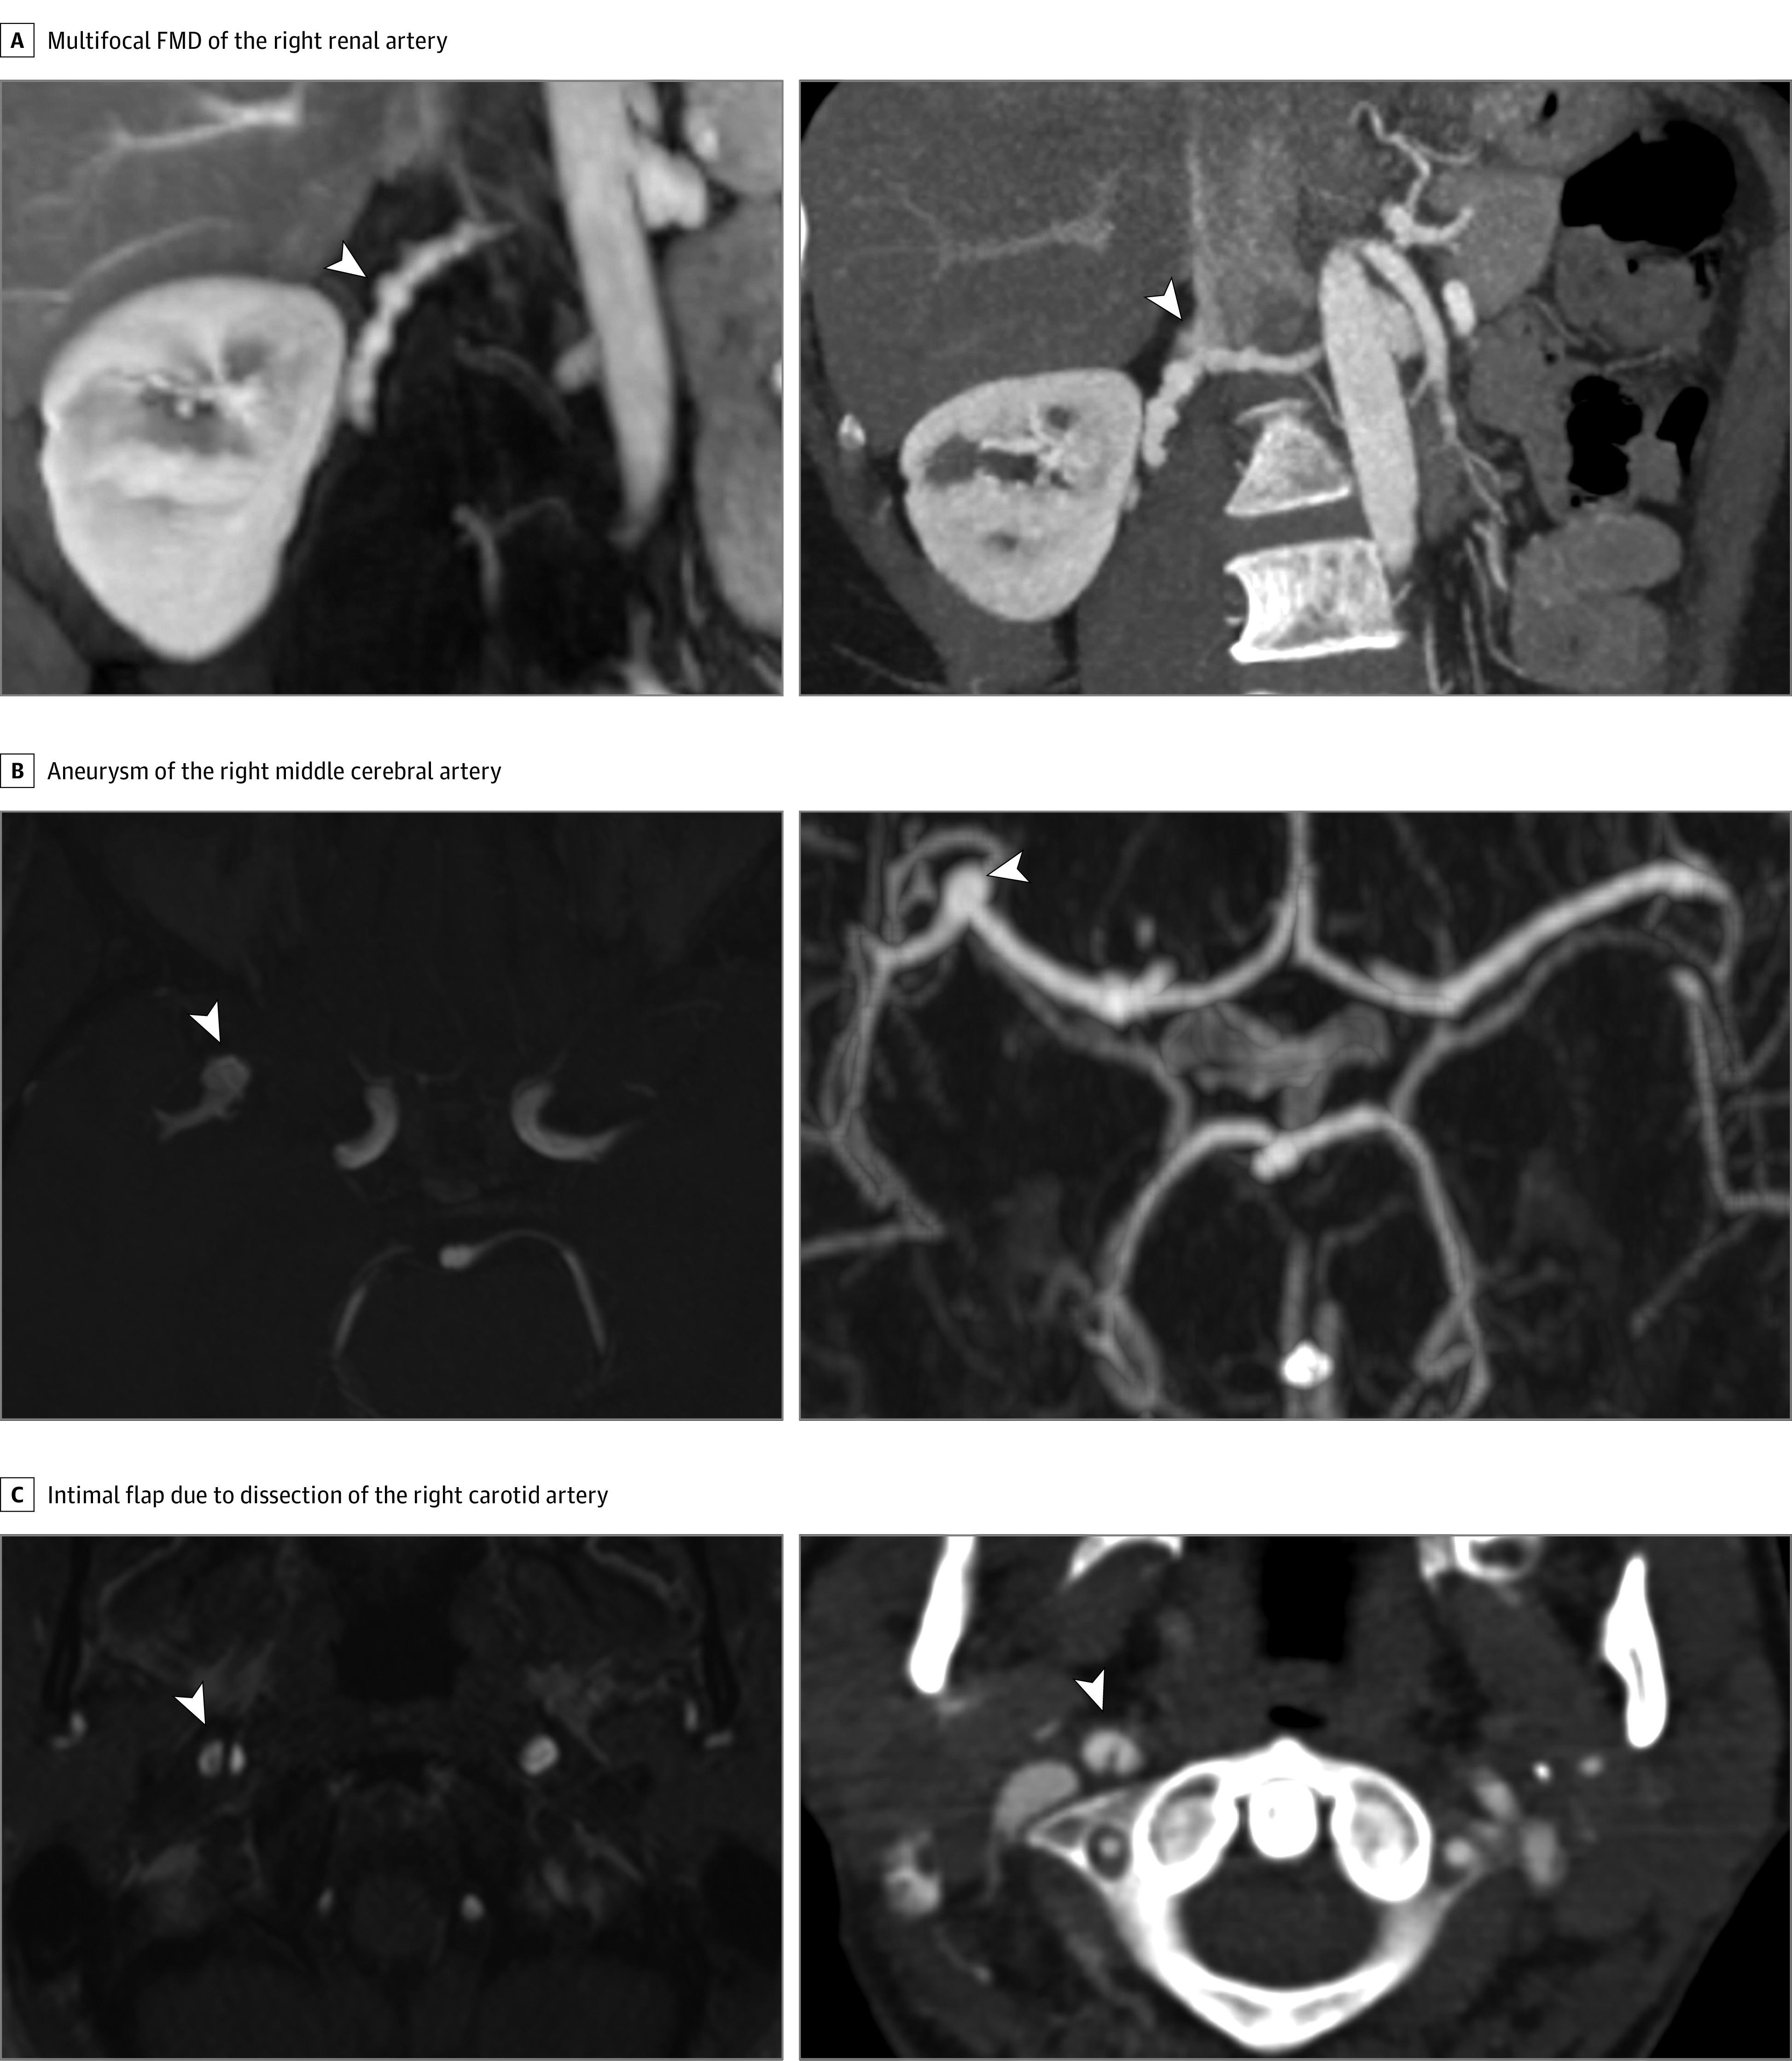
Examples of Extracoronary Arterial Abnormalities in 3 Patients With Spontaneous Coronary Artery Dissection Visualized by Computed Tomographic Angiography (CTA) and Magnetic Resonance Angiography (MRA) A, Multifocal fibromuscular dysplasia (FMD) (string of beads) of the mid-distal segments of the right renal artery. B, Aneurysm of the right middle cerebral artery (M1-M2 junction). C, Intimal flap due to a dissection of the right carotid artery. Lesions are indicated by arrowheads.
